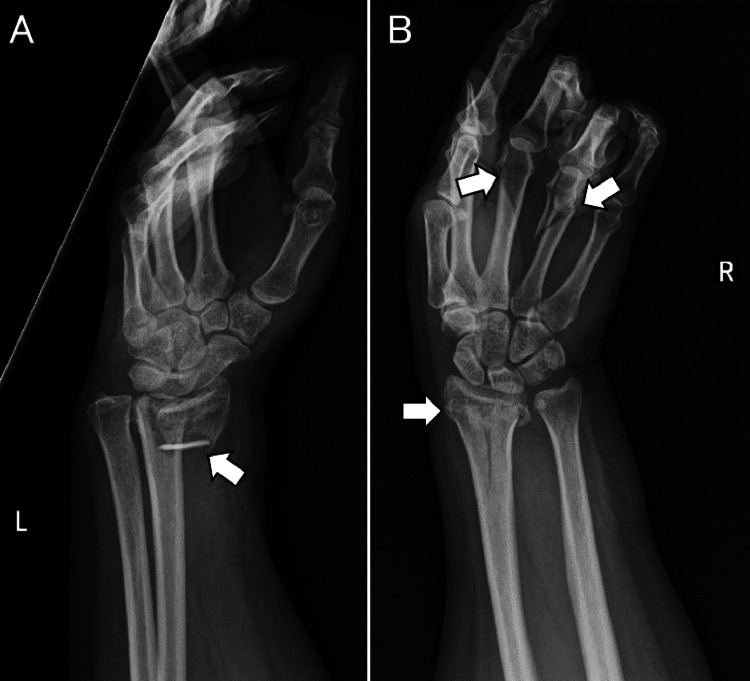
Plain radiographs of the upper extremities. Left wrist radiograph demonstrating a distal radius fracture (arrow) (A). Right hand and wrist radiograph demonstrating fractures of the third and fourth metacarpals and a distal radius fracture (arrows) (B).
